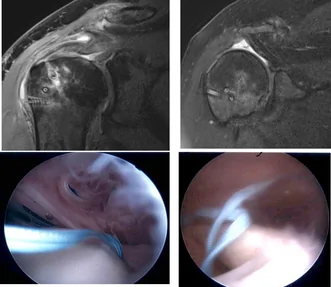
Magnetic resonance imaging: T2‐weighted sagittal images showing a rotator cuff repair failure with suture limbs in continuity with the tendon. Intraoperative images: posterior view of the subacromial space of 2 separate patients showing rotator cuff repair failure caused by suture slippage from a lateral row anchor.
